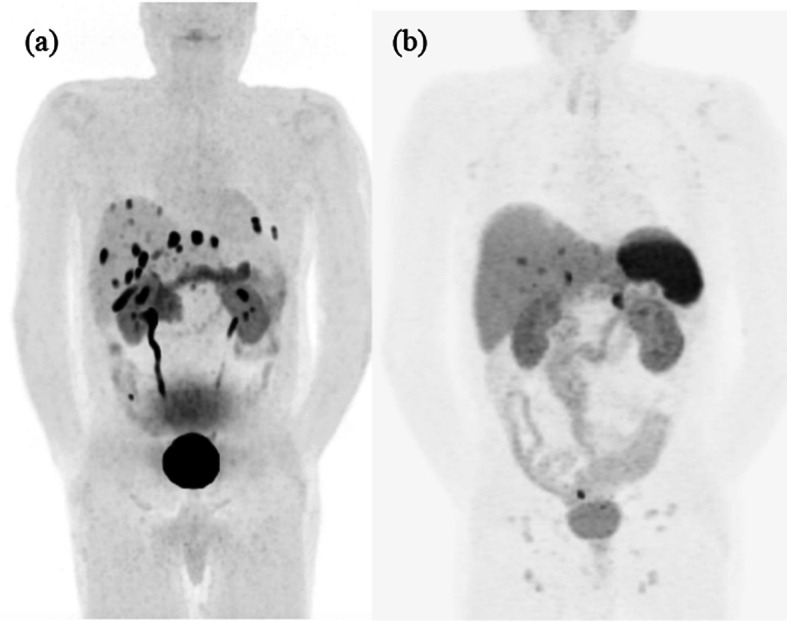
18F-DOPA PET/CT MIP (a) and 68Ga-HA-DOTATATE PET/CT MIP (b) performed 22 days apart. While some metastases were DOTATATE-avid, the 18F-DOPA scan revealed many more sites of discordant non-DOTATATE-avid disease
